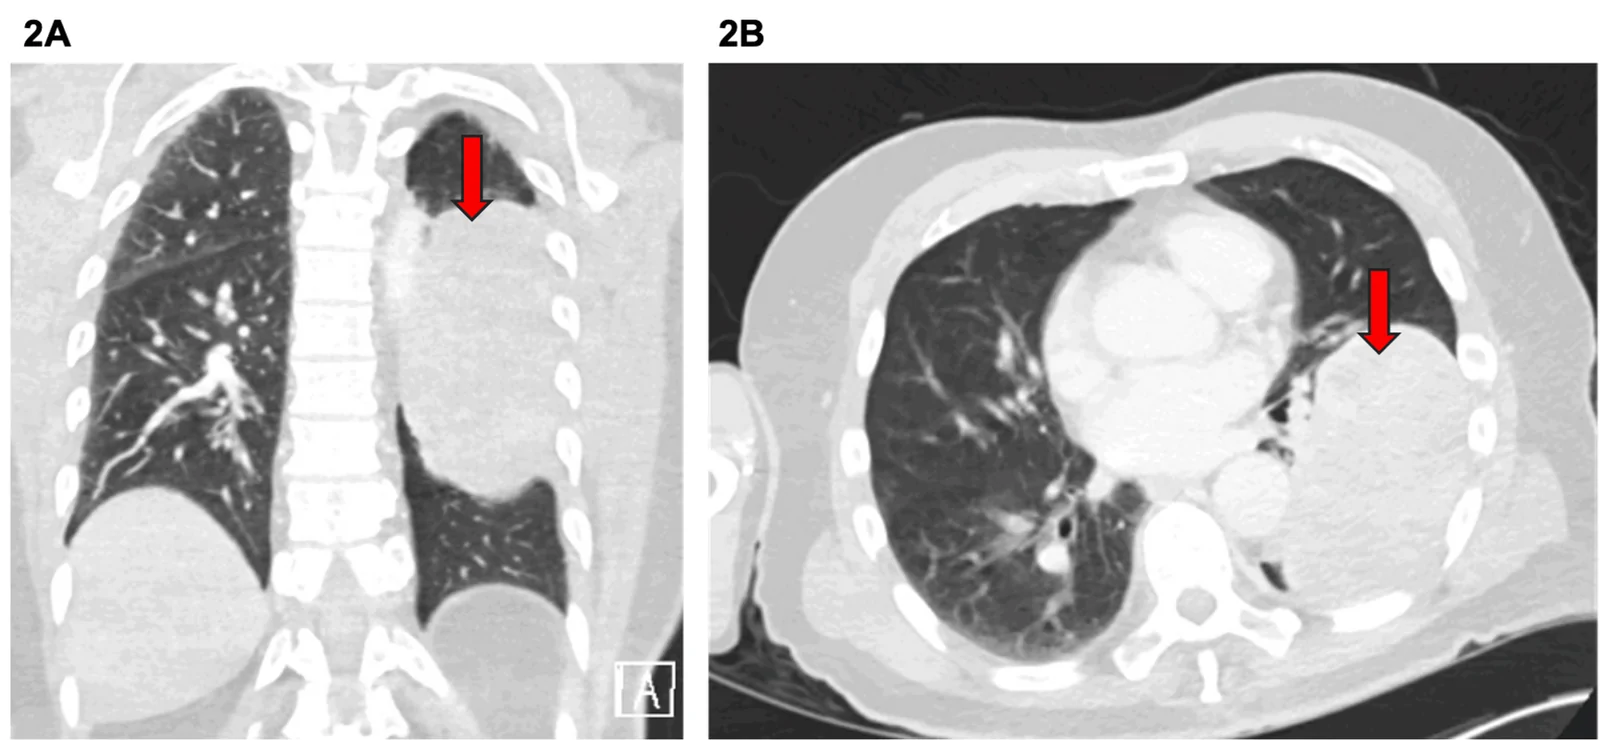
CT of the chest demonstrating a 12.5 × 10.4 × 6.5 cm well-demarcated soft tissue tumor in the left lower lobe without pleural effusion, pleural thickening, or pneumothorax, with incidental peripheral filling defects within the pulmonary arterial branches of the left upper lobe and right lower lobe suggestive of pulmonary embolism
